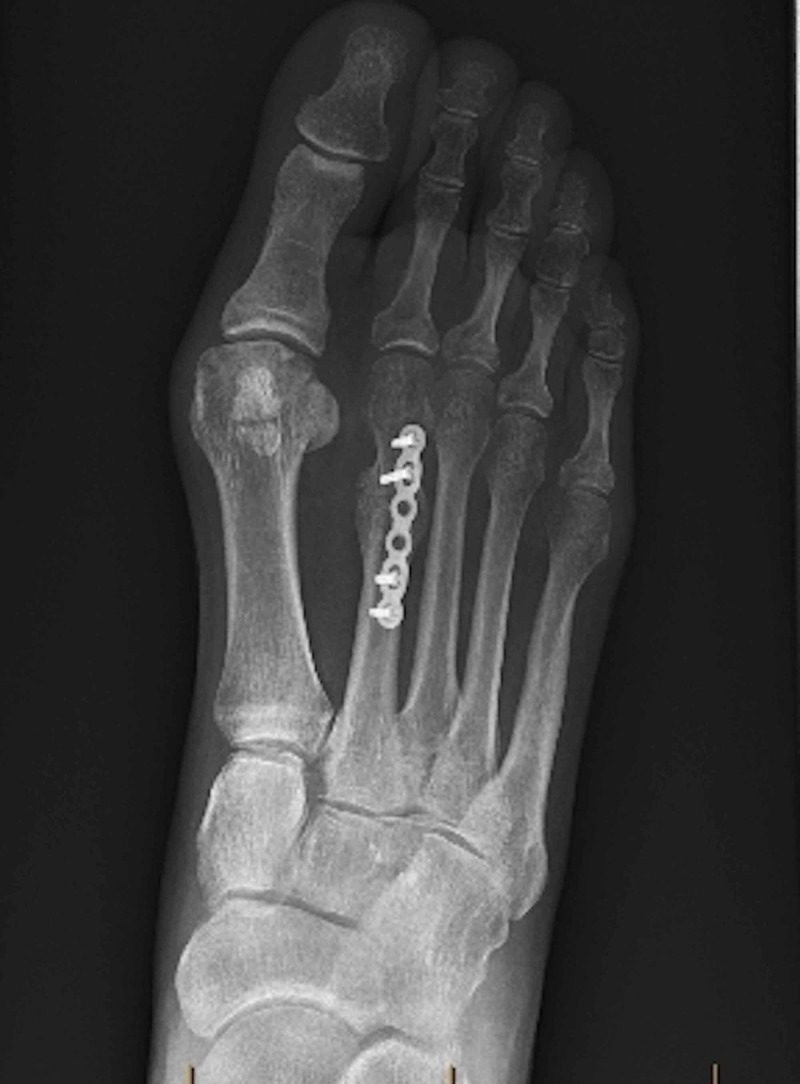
Post-operative anterior-posterior radiograph showing ORIF with re-establishment of the metatarsal parabola ORIF, open reduction with internal fixation
